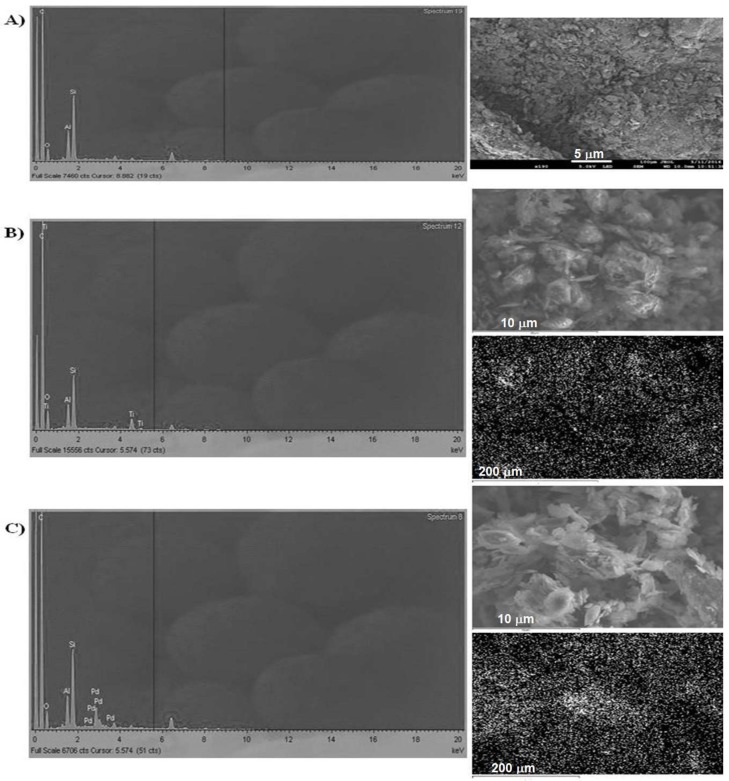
Results for SEM and energy-dispersive X-ray (EDX) characterization of: graphite powder (A), and carbon nano-modified powder with titanium (B) and palladium (C) oxide particles.
